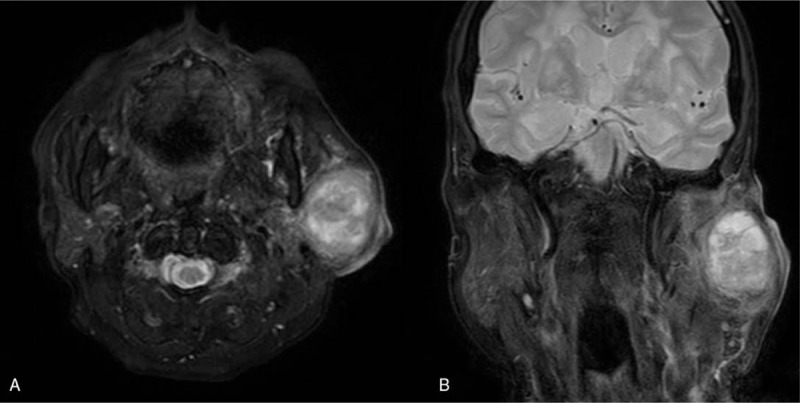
MRI revealing a necrotic mass in the superficial lobe of the left parotid gland in T2-weighted images in axial view (A) and coronal view (B). MRI = magnetic resonance imaging.
